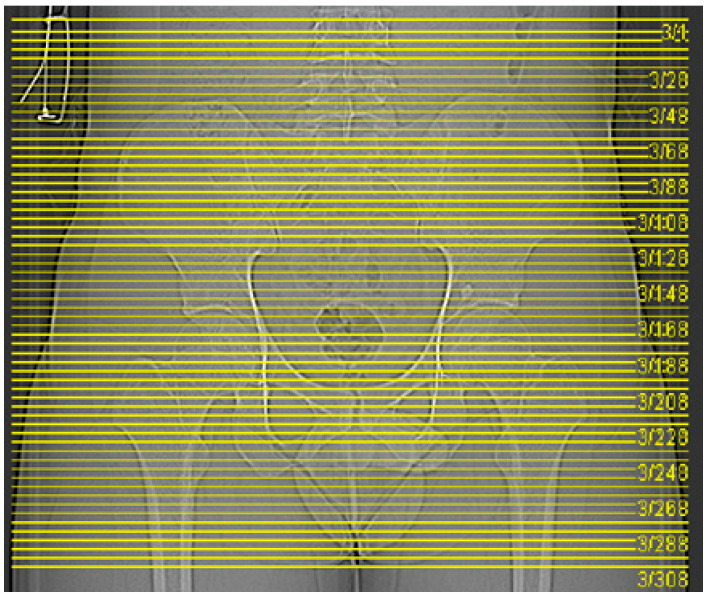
A pelvic CT scan of a healthy adult.
